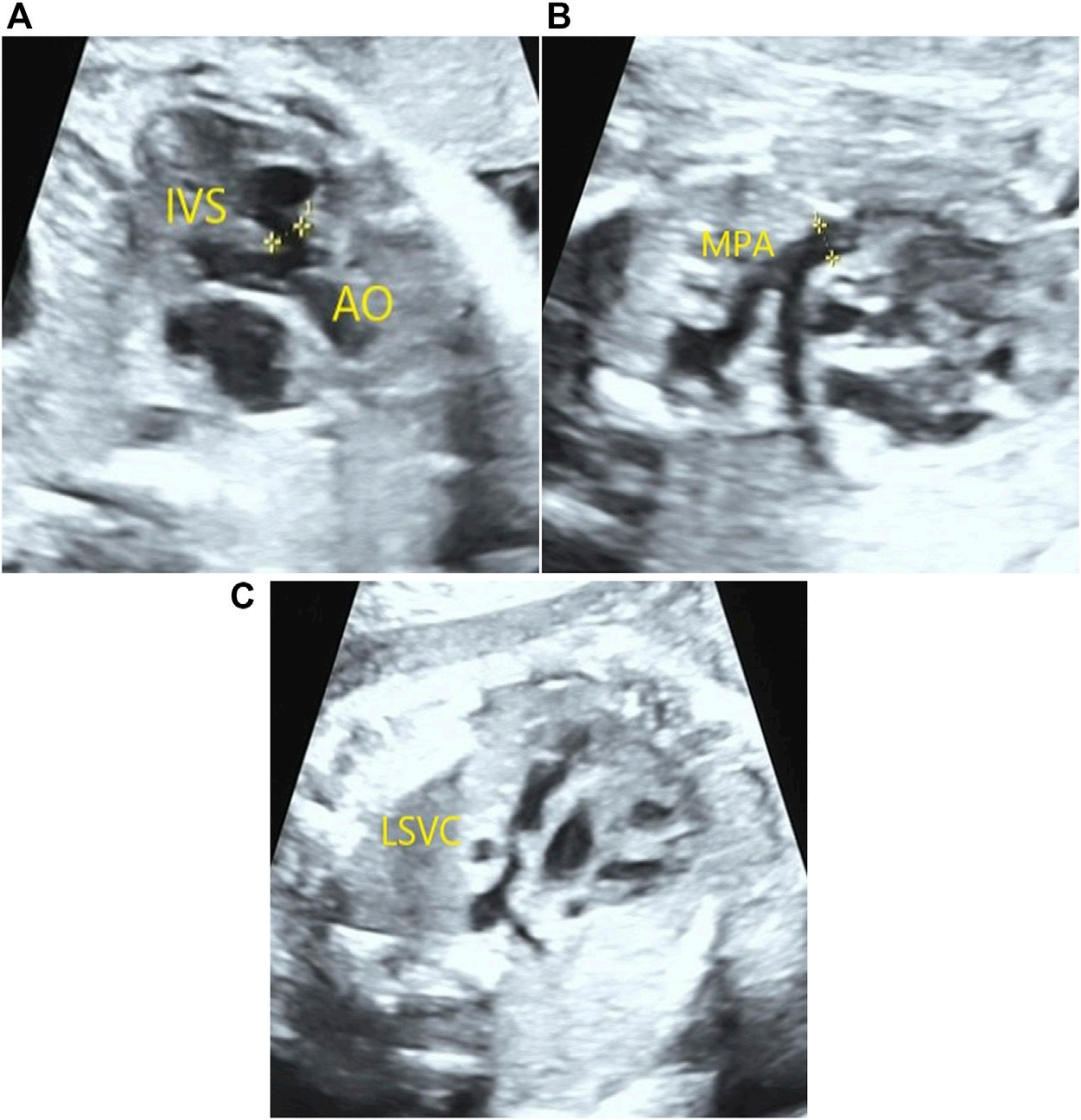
Congenital heart defects detected in the fetus by prenatal ultrasound examination. A 0.41-cm ventricular septal defect and overriding aorta (A) were detected in the fetus by prenatal ultrasound examination. In addition, pulmonary artery stenosis (B) and persistent left superior vena cava (C) were also observed.
